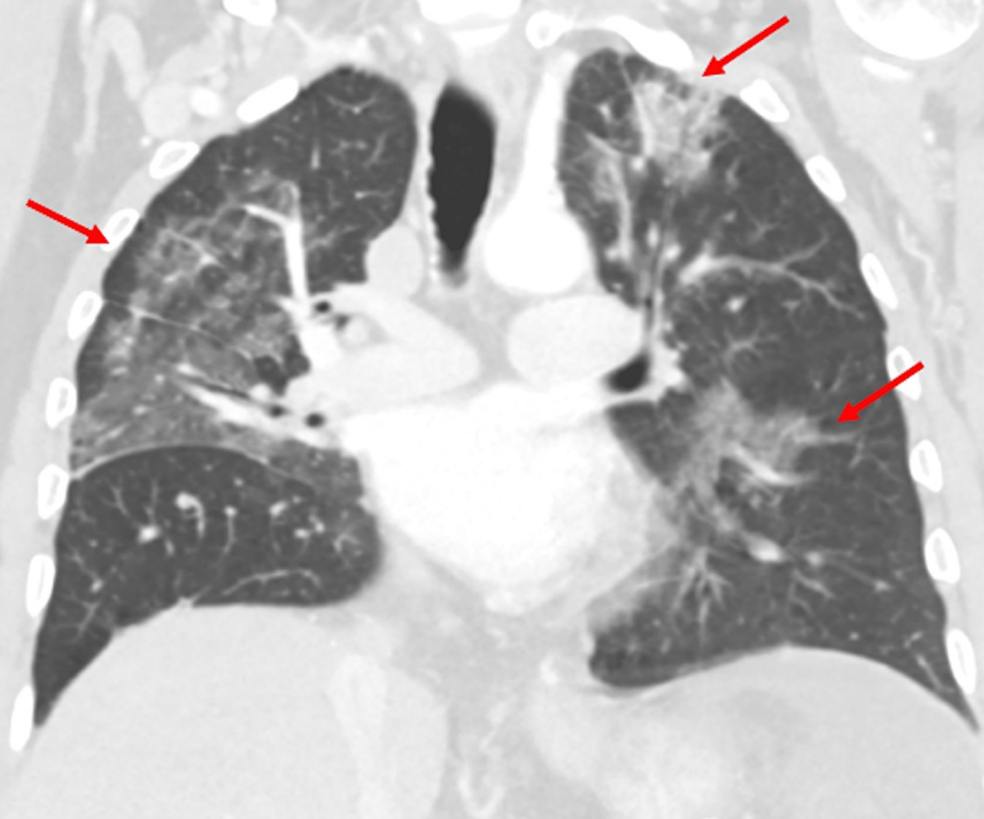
Coronal CT of the chest showing bilateral ground glass opacities Chest CT shows the progression of bilateral multifocal ground glass consolidation (red arrows) in both lungs representing the progression of multifocal pneumonia.
